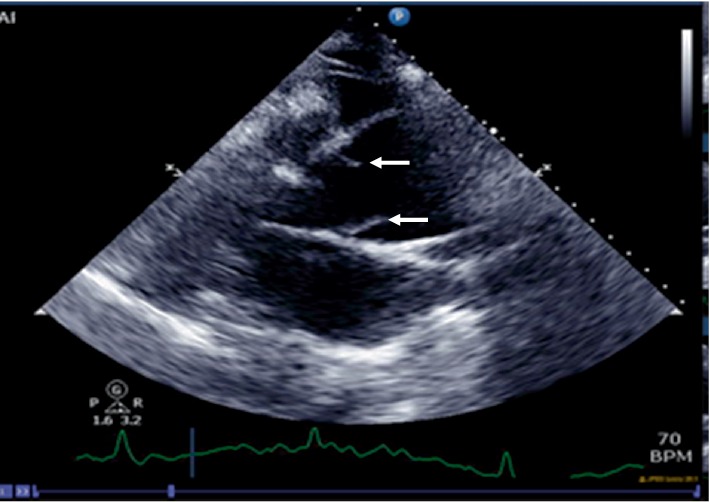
Parasternal long axis view showing the dissection flap (arrows) in the ascending aorta.
